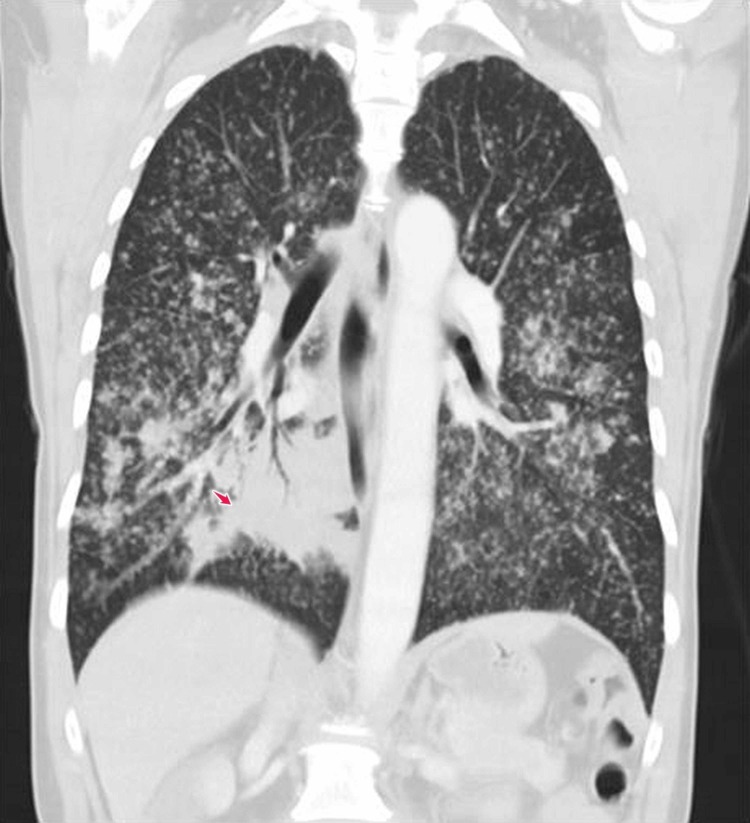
CT scan chest Computerized tomography (CT) scan of chest with a mass-like prominence in the right lower lung field (arrow)
